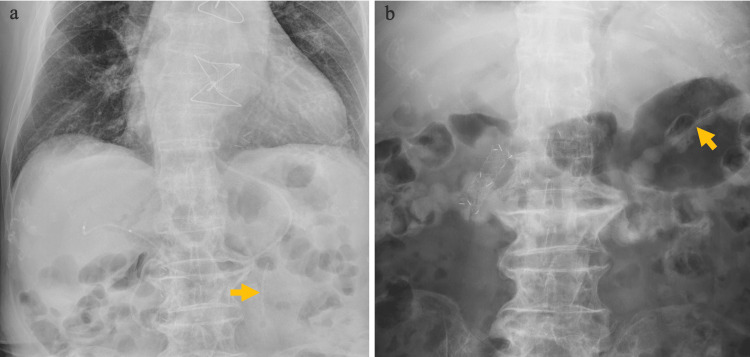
Abdominal X-ray images showing uncomplicated progression of the PSDS X-ray images taken on the 10th (a) and 12th (b) days confirmed an uncomplicated progression of the PSDS (yellow arrows) through the intestinal tract. PSDS, pancreatic spontaneous dislodgement stent
